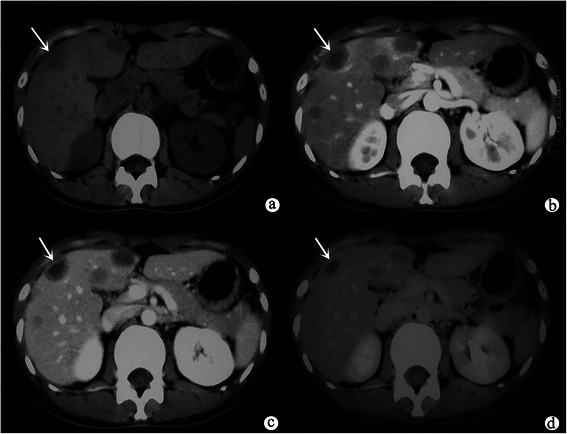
Axial unenhanced CT (a) showed that multiple nodular lesions (indicated by white and black arrow) were homogeneous and hypodense in the liver. Capsular retraction also could be seen (indicated by black arrow). Axial arterial phase (b) showed the lesion (indicated by the white arrows) was ring-like enhancement. Axial portal vein phase (c) showed the lesion (indicated by white arrow) was progressive enhancement. Axial delayed phase (d) the lesion (indicated by white arrow) was progressive enhancement
